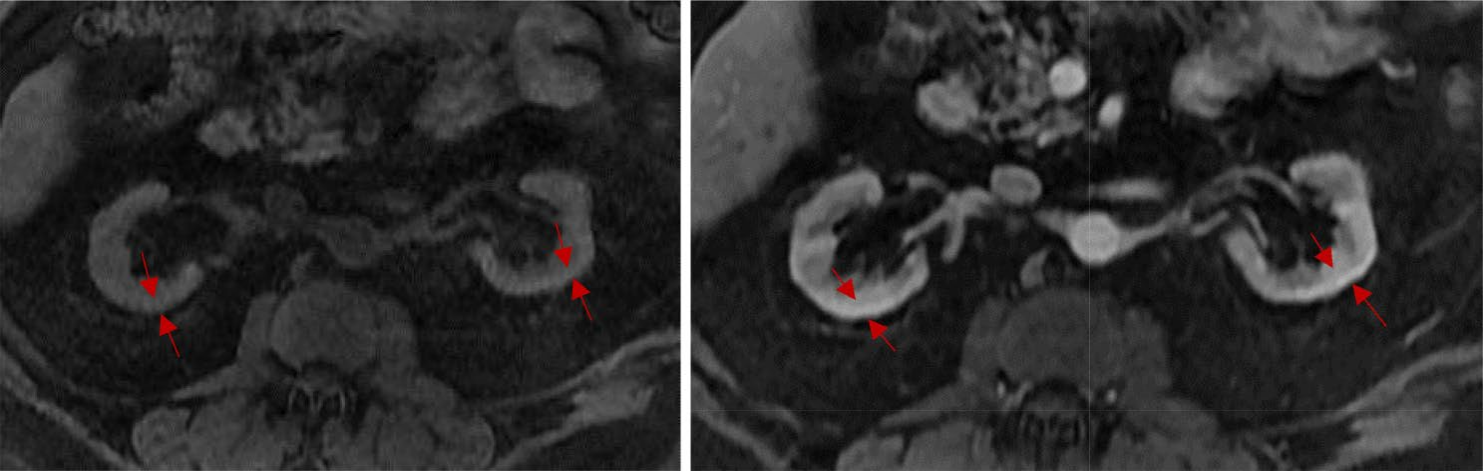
Renal MRI. Pre and post T1 show homogenous, symmetric renal cortical thinning (red arrows)
